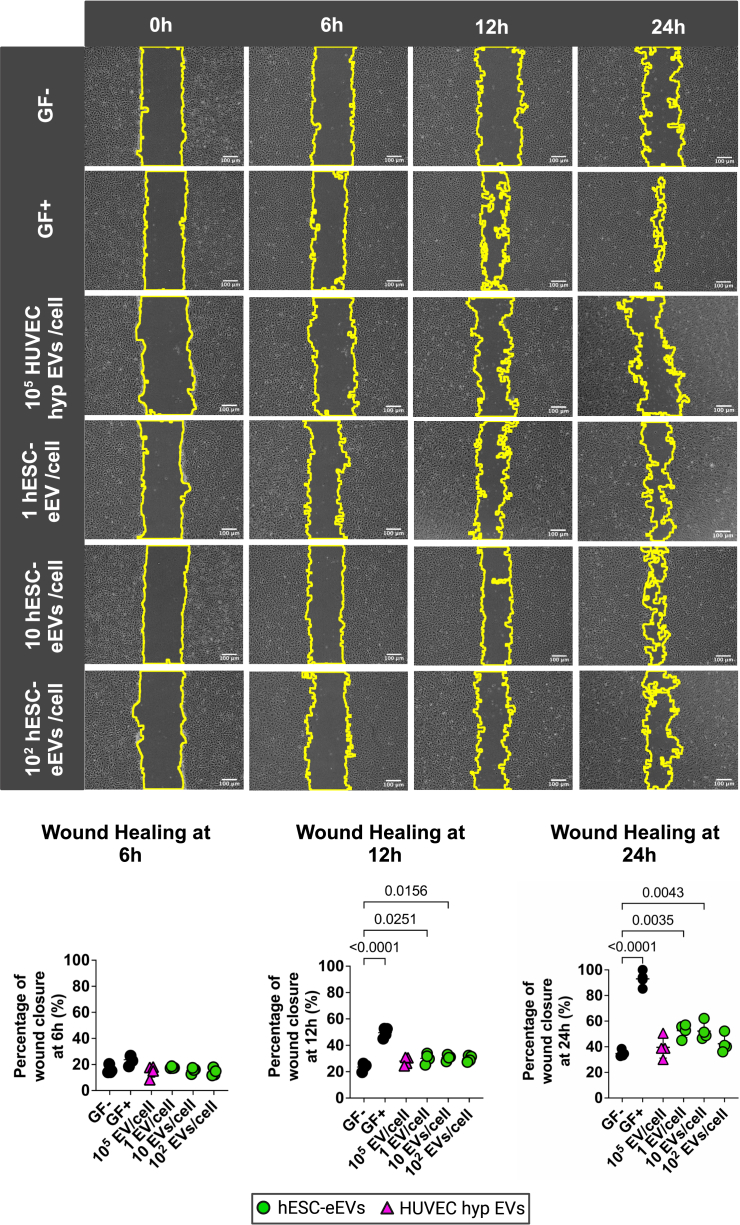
EVs from hESC-ECPs promote EC wound healing at low concentrations Wound healing assay on HCMECs using increasing concentrations of EVs from hESC-eEVs (dose range 1–10^2^ EV/cell) or treated with 10^5^ HUVEC hypoxic EVs/cell (n = 4). Cells cultured in basal media and treated with sterile filtered PBS (vehicle control) served as negative control (GF^−^). Cells in fully supplemented media (GF^+^) served as positive controls. Graphs (below) represent the percentage of wound closure at 6, 12, and 24 h postwound induction. Data were analyzed using the MRI wound healing tool. The control samples (GF+ and GF−) are depicted as black circles, the HUVEC hypoxic EV treated samples (HUVEC hyp EVs) are depicted as purple triangles, and the hESC-eEV-treated samples are depicted as green circles. Statistical significance (indicated with p values) was determined by 1-way ANOVA with Dunnett’s multiple comparisons test. Error bars represent the SD. Scale bar, 100 μm.
